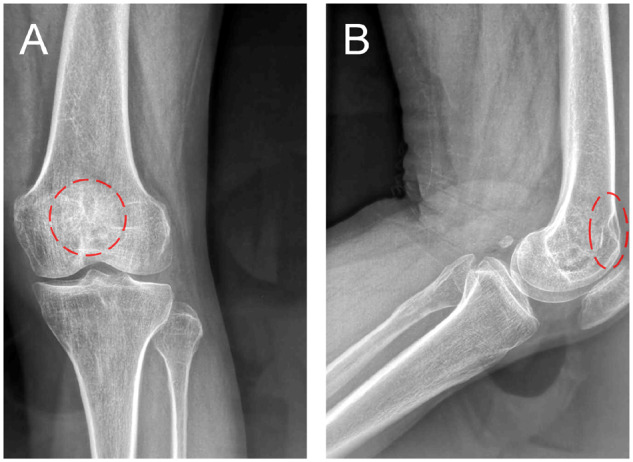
Frontal (A) and lateral (B) views of plain radiography at 12 months after the operation (the surgical site is noted by dashed circle in each picture).
